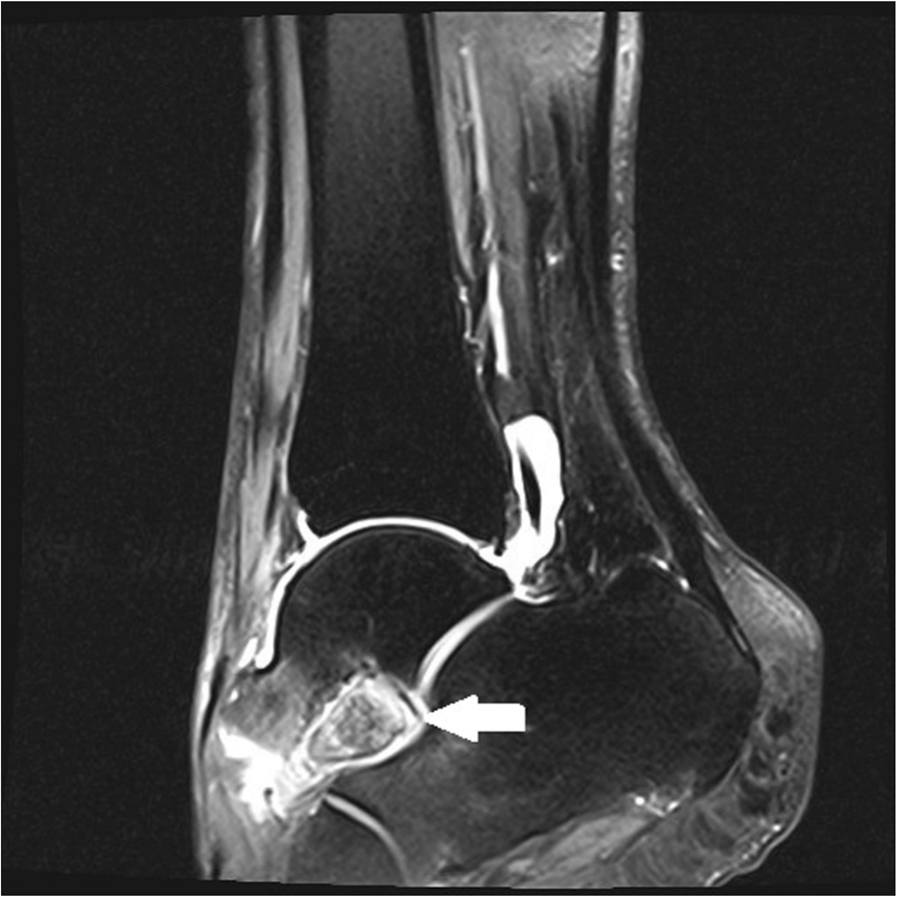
Sagittal magnetic resonance imaging of the foot, T2-weighted image showing the calcaneus secundarius (arrow) preoperatively.
